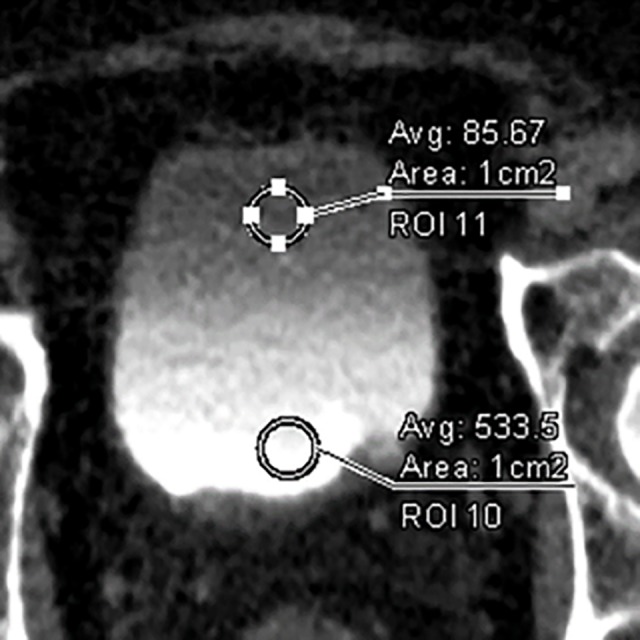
Axial CT images obtained in the excretory phase with a 5-minute delay in a 67-year-old man, with ROIs manually drawn at the anterior and posterior portions of the urinary bladder. The sizes of the ROIs were kept constant on the anterior and posterior portions with a copy-and-paste function.
